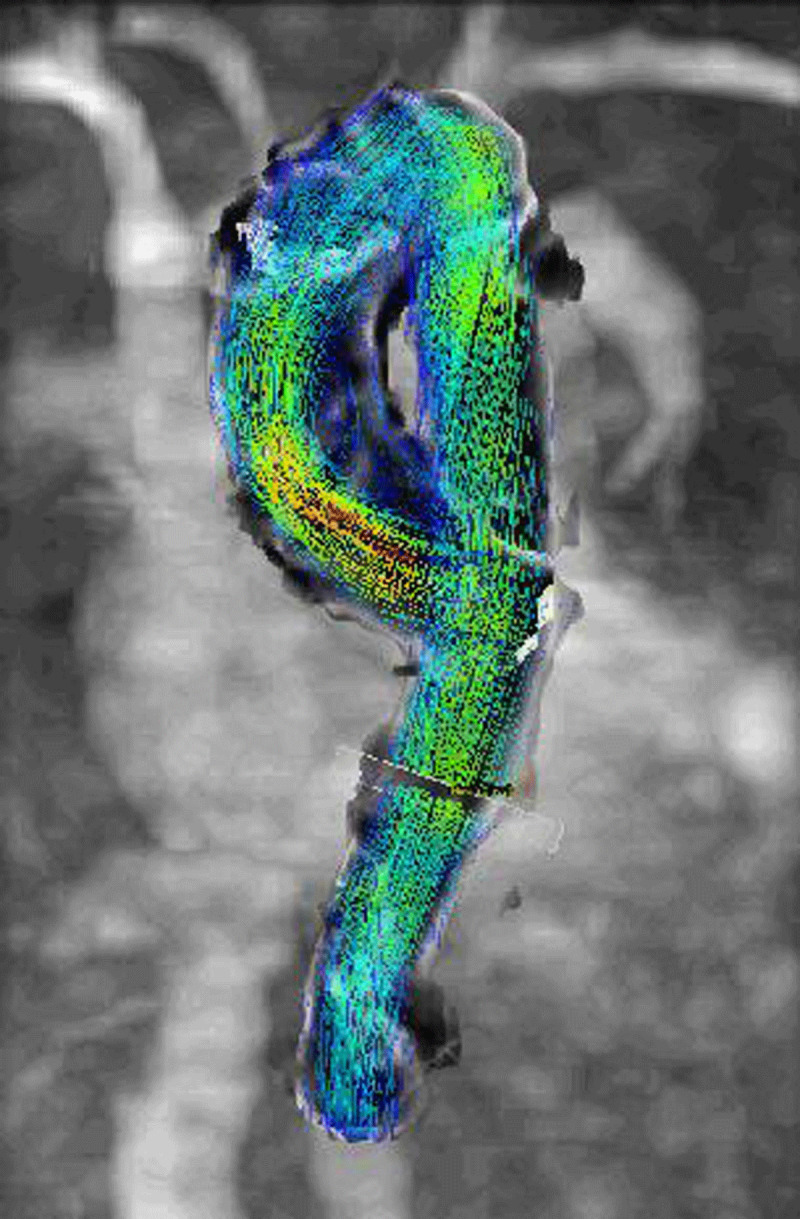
An example of four-dimensional (4D) flow magnetic resonance imaging, which enables the visualization of the temporal evolution of complex blood flow patterns within an acquired 3D volume. The blood flow patterns are represented in the colored vectors, which represent velocity with blue being closer to 0 m/s and red up to 1 m/s.^17,18,19^
